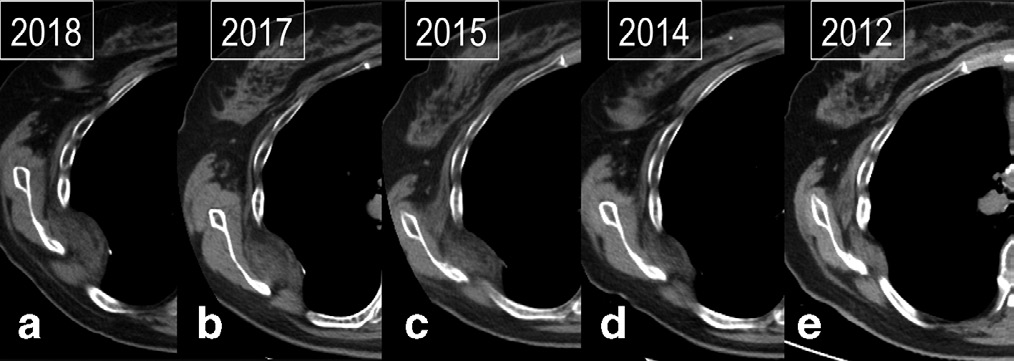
(a–e) Serial CT images showing progressive enlargement of chest wall mass over 6 years.
